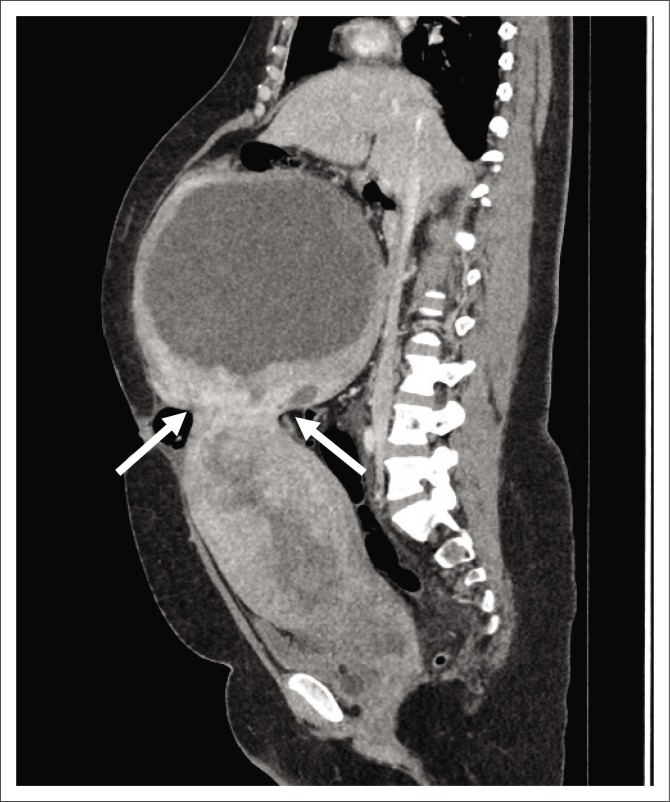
Sagittal portal venous computed tomography shows that the mass is connected to the uterine fundus (arrows).
